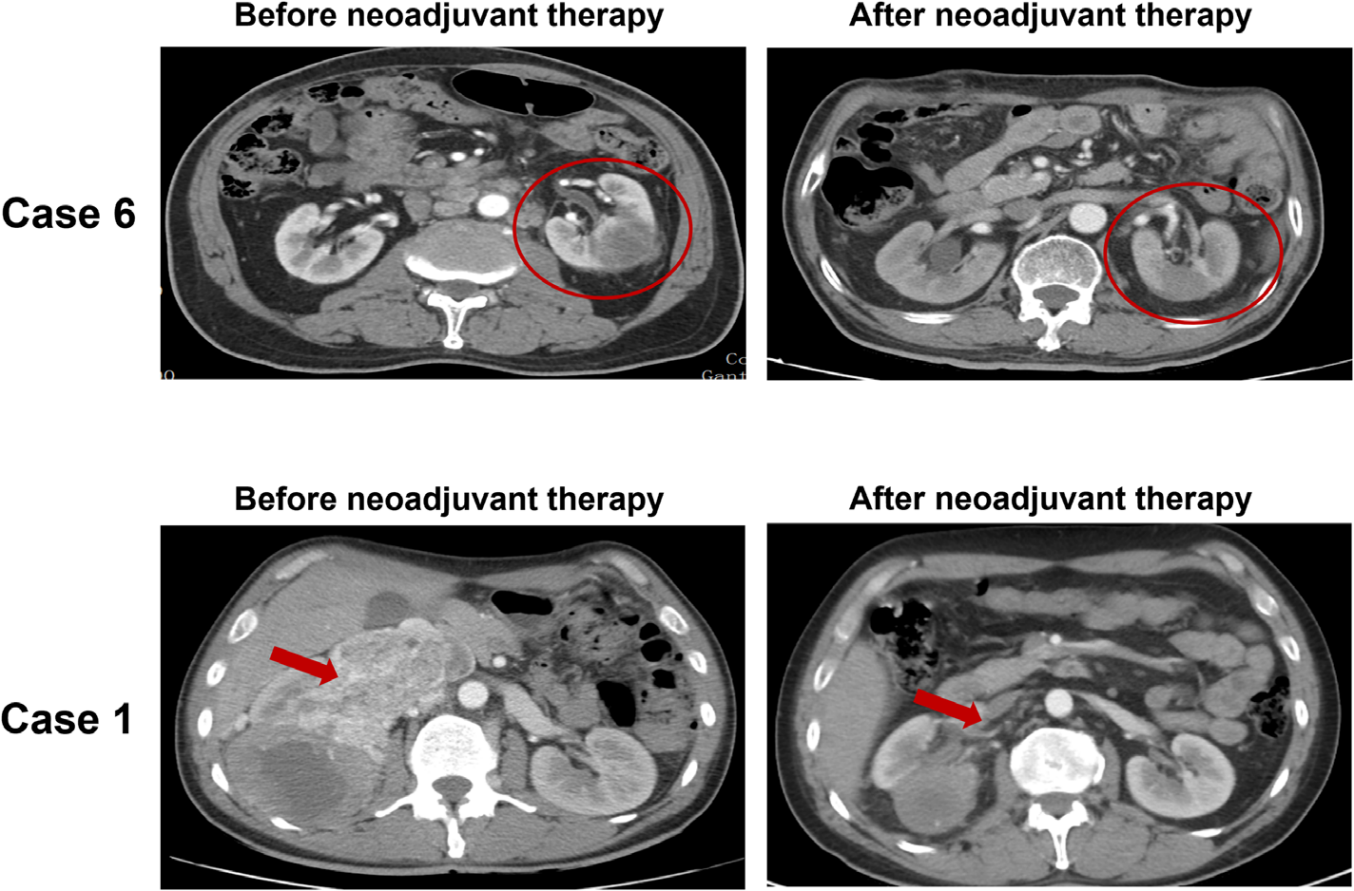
Changes in tumour thrombus and primary renal tumours in cases 1 and 6. Left: CT scan or MRI before the neoadjuvant therapy. Right: CT scan or MRI after the neoadjuvant therapy. The red circle indicates the primary renal tumour. The red arrowhead indicates the venous tumour thrombus.
